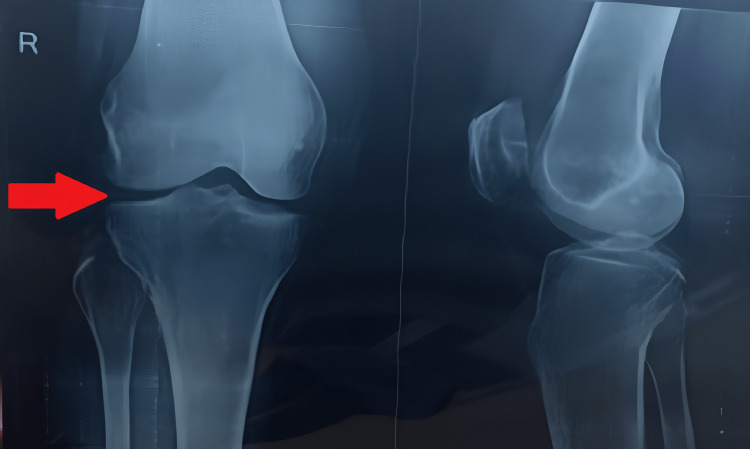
Right knee X-ray revealing injury to the lateral tibial plateau (red arrow)
